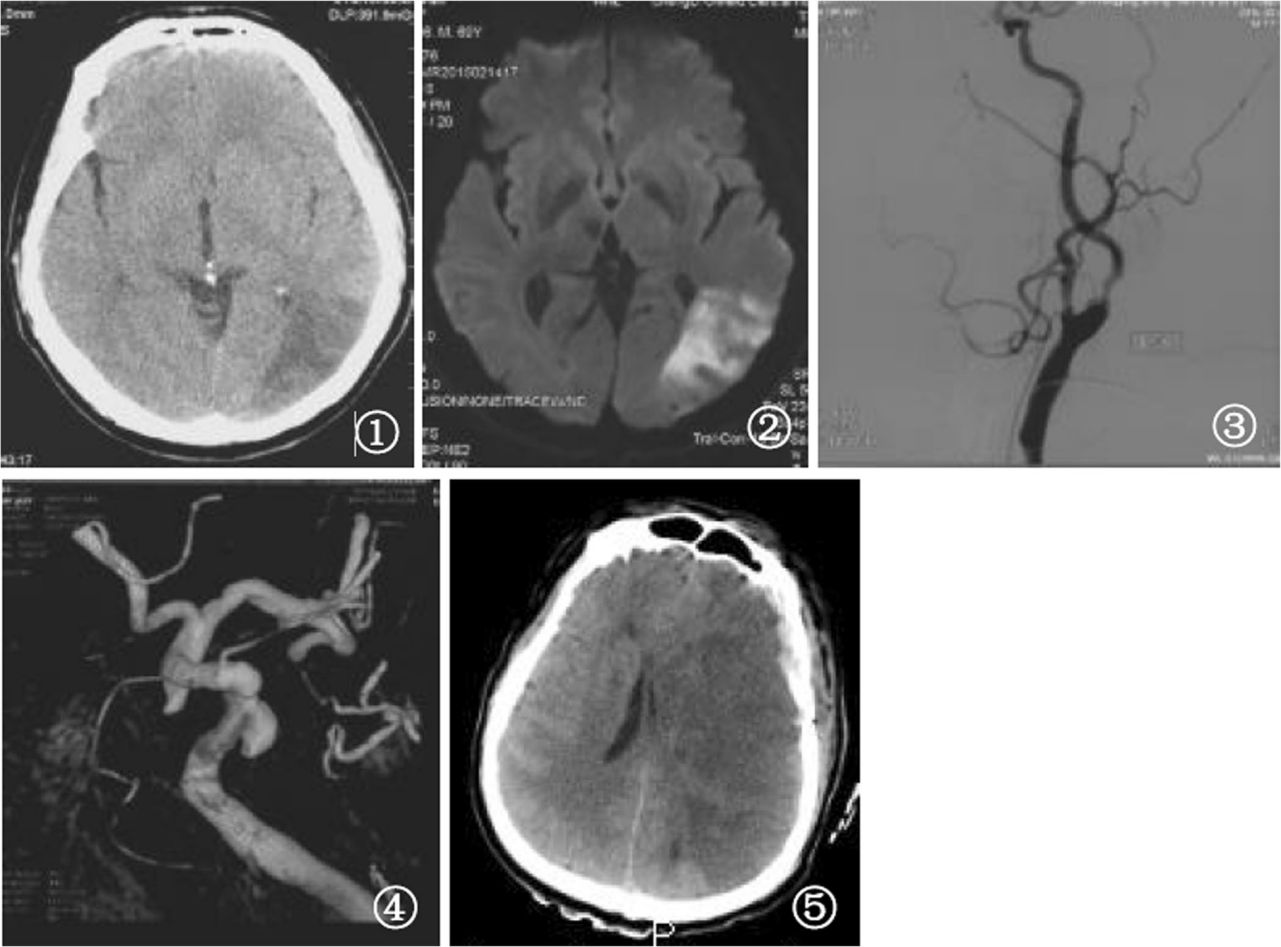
One patient, 65–70 years old. ① cerebral infarction (left occipital lobe) shown in Computed Tomography scan before surgical operation; ② cerebral infarction (left occipital lobe) shown in Magnetic Resonance Imaging before surgical operation; ③left carotid arterial stenosis (> 75%) shown in Digital Subtraction Angiography before surgical operation; ④ left posterior communicating aneurysms with a diameter of 5.6 mm shown in Digital Subtraction Angiography before surgical operation; ⑤ cerebral infarction (left hemisphere) with a large area shown in Computed Tomography scan after operation
